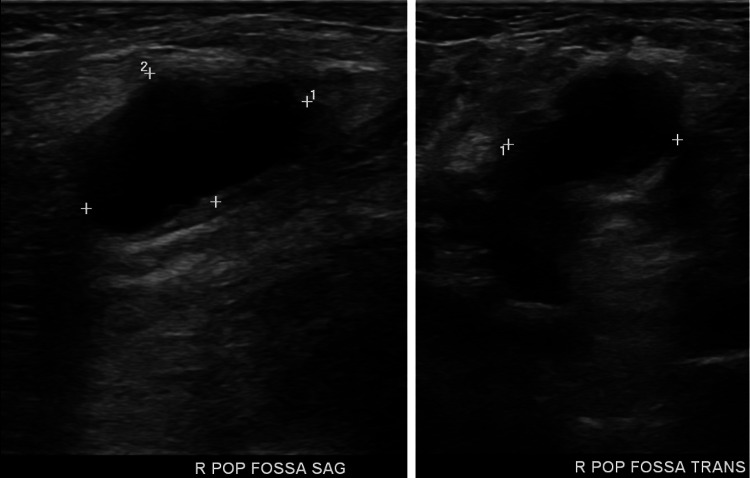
Ultrasonography of lower extremity reveals a popliteal cyst (crosshatches)
